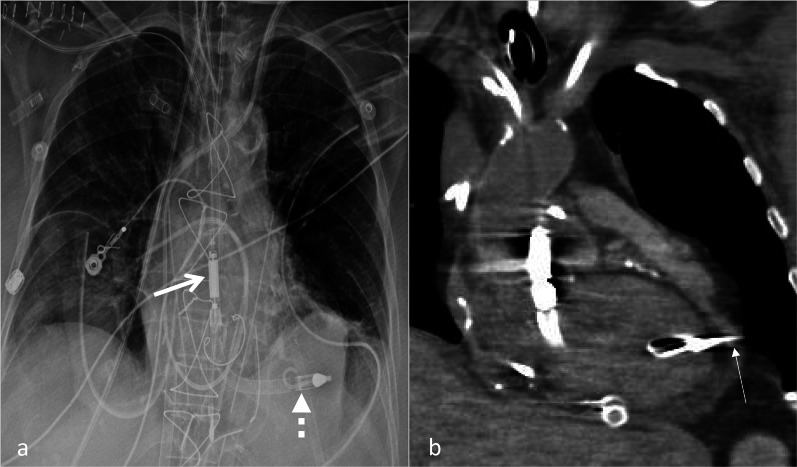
Complication from Impella. AP Chest radiograph with a left ventricle Impella device (a). The inlet port of the Impella is not in expected location on this radiograph, outlet port is much below the carina (white arrow) and the pigtail portion is coiled in the left ventricle cavity (white dashed arrow). The pigtail portioned is coiled, this is a sign of malposition. This can indicate entrapment within the papillary muscle apparatus. Non-contrast coronal CT at the level of the LVOT (b) confirms low positioned of the Impella device. The tip of the device has perforated the left ventricular wall (white arrow) and high density fluid can be seen in the pericardium, compatible with hemopericardium. (LVOT—Left ventricle outflow tract)
